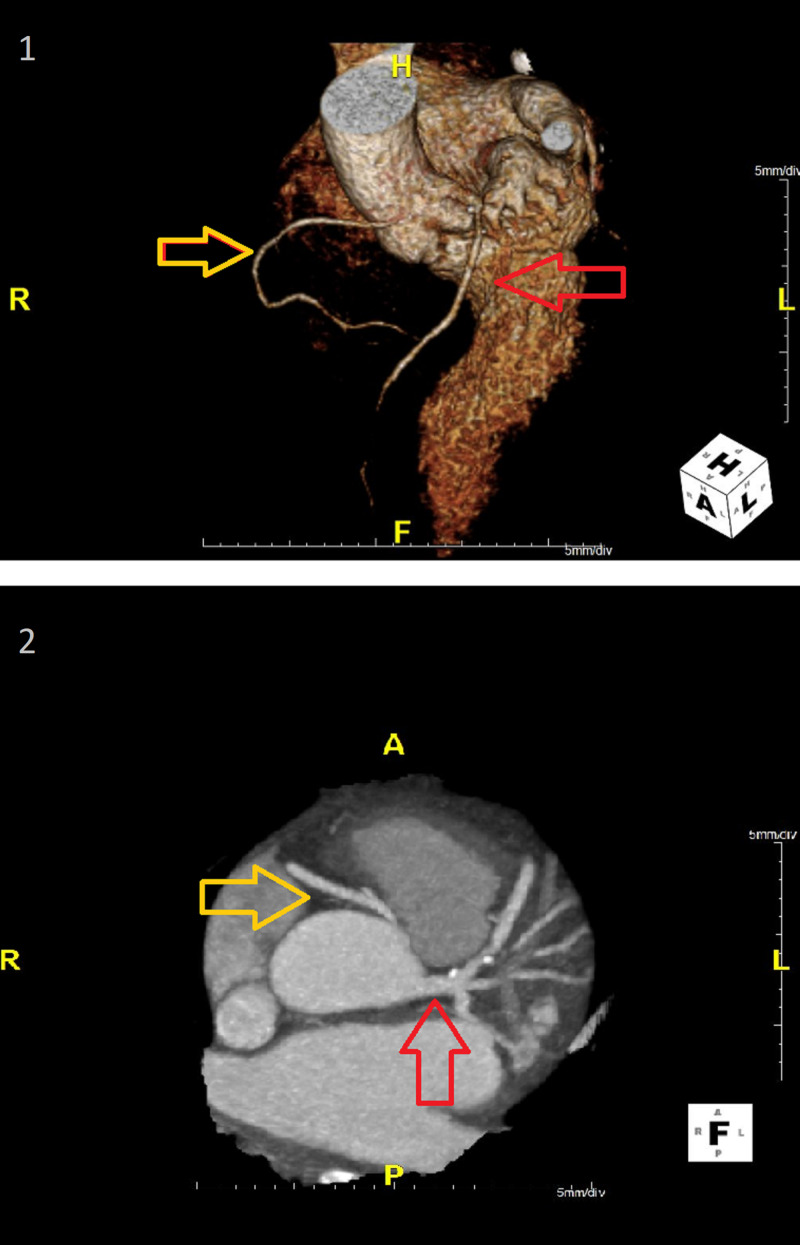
CT scan volume-rendered reconstruction (1) and oblique maximum intensity projection (2) of the coronary arteries. The yellow arrows designate the right coronary artery, and the red arrows the left coronary artery. Both arteries arise from the left coronary sinus.
